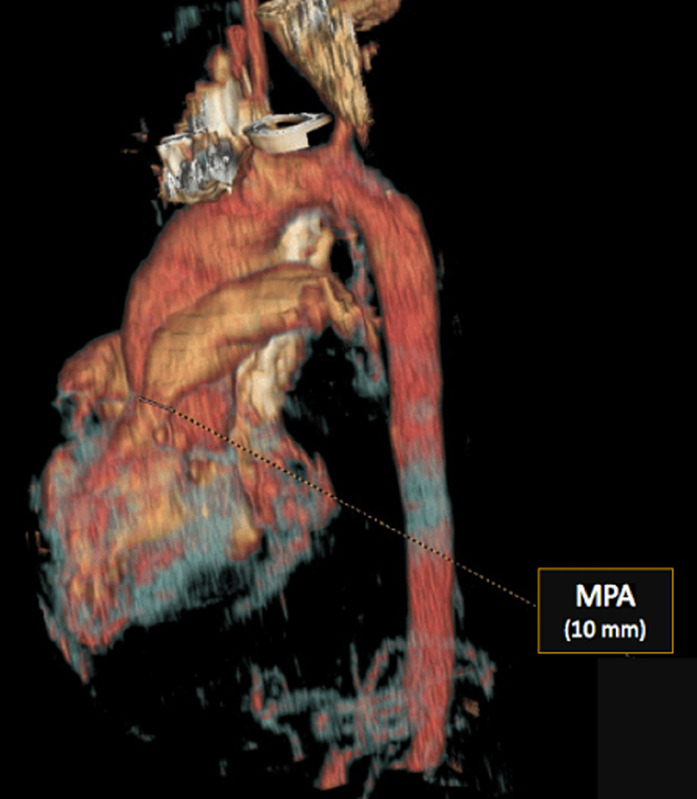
3D contrast-enhanced computed tomography image demonstrating hypoplastic main pulmonary artery
